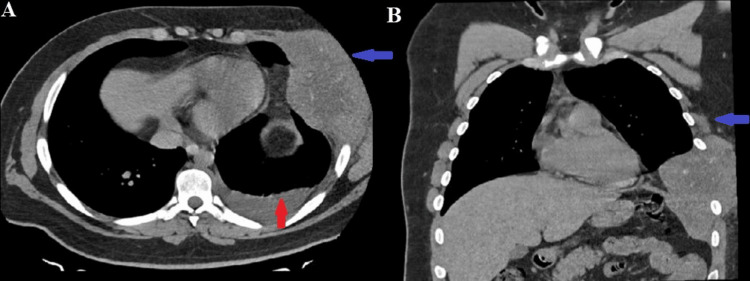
(A and B). A native CT scan of the lung in the axial and coronal plane shows a tumor formation (Ewing's sarcoma) located on the left in the region of the 6th rib, along the mid-axillary line, with the presence of a soft tissue lesion, destruction of the adjacent rib. Propagation to the lung, with infiltration of the pleura and chest wall, dimensions 15-10 cm (blue arrow). A small pleural effusion on the left (red arrow).
